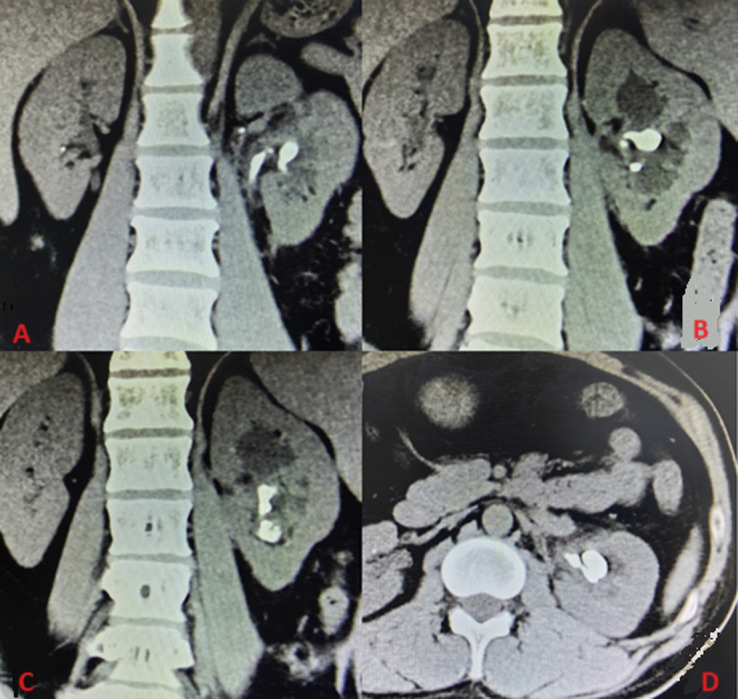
computed tomography showing ureteral stent encrustation and kidney stones; A,B) ureteral stent and ureteral stent encrustation; C) kidney stones located in the renal pelvis and subrenal calyx; D) kidney stones of CT cross-sectional imaging
